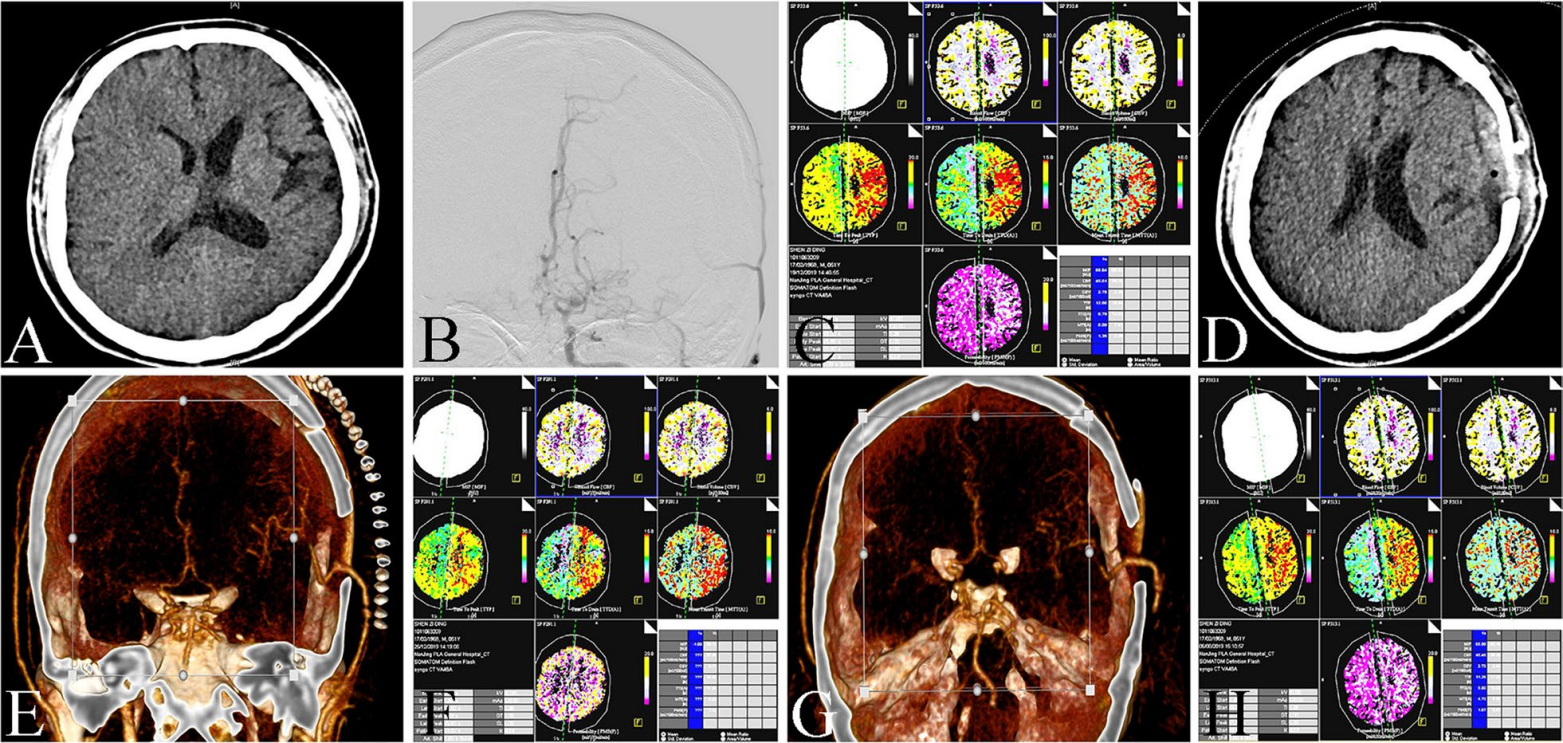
The image data of STA-MCA bypass. A Head CT showed infarcts in the left frontal lobe, corona radiata and centrum ovale. B DSA showed occlusion in left ICA. C CTP showed that the perfusion of left hemisphere was lower than that of right hemisphere. D Head CT showed that there was no new infarction or bleeding in the operation area. E CTA showed that the bypass vessels were unobstructed. F CTP showed that the left hemisphere perfusion was better than that before operation. G CTA showed that the bypass vessels were unobstructed after three months of follow-up. H CTP showed that left hemisphere perfusion was further improved after three months of follow-up
